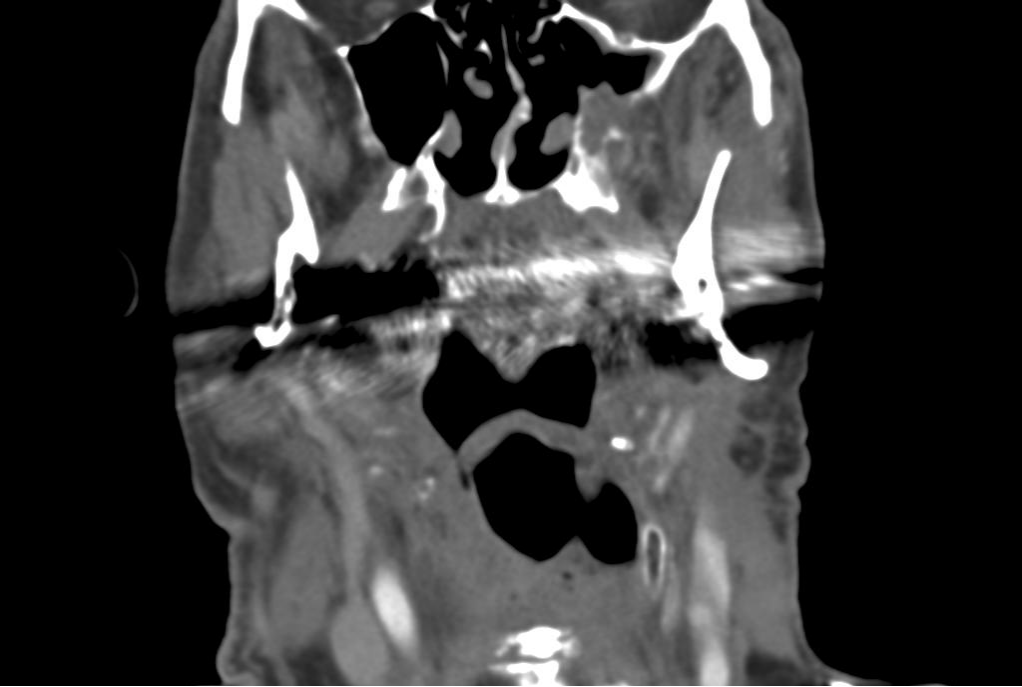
Lymph node metastasis Level II detected by CT-scans.
